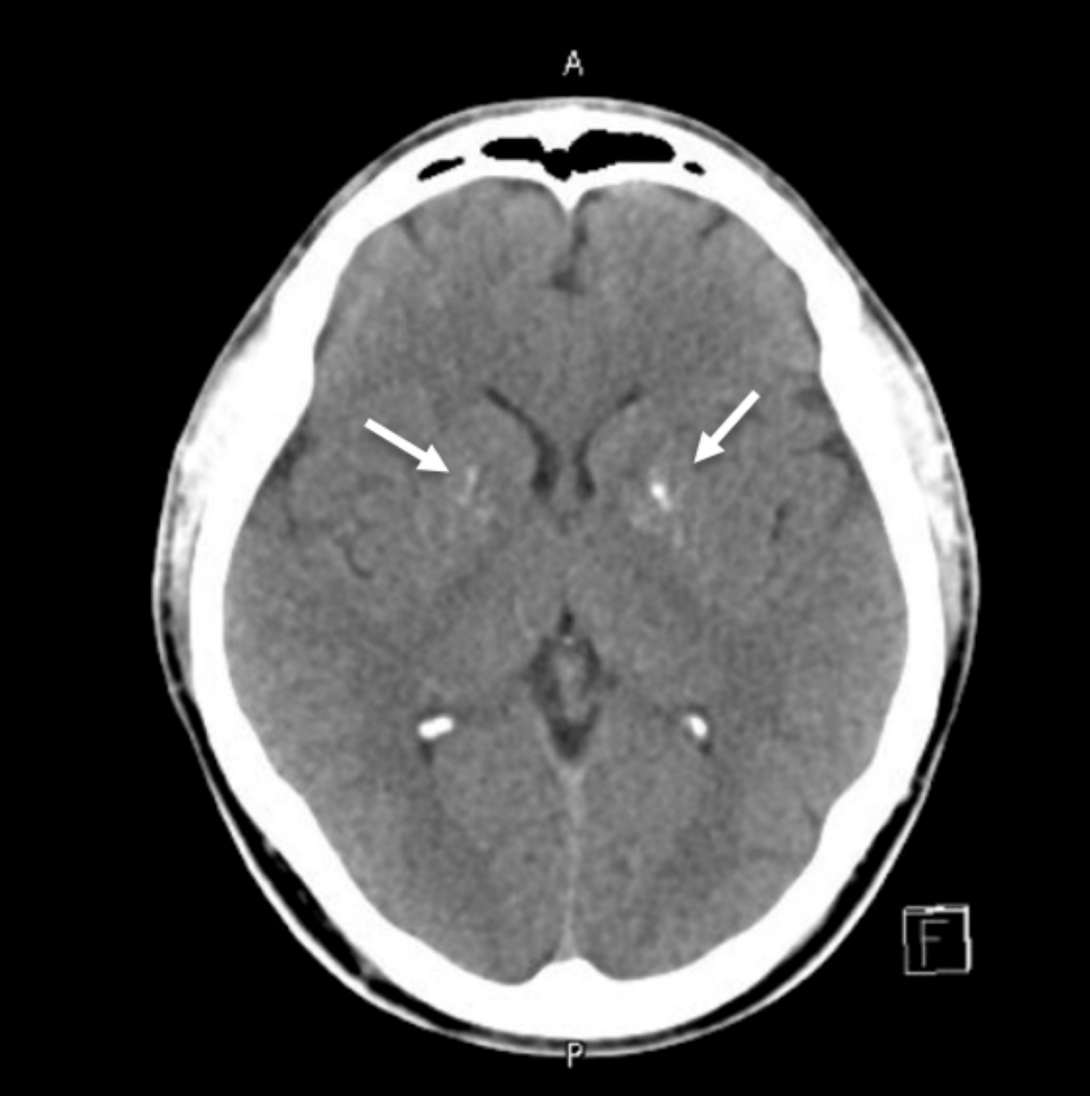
CT head demonstrating bilateral basal ganglia calcifications. White arrows point to the areas of bilateral basal ganglia calcifications.
